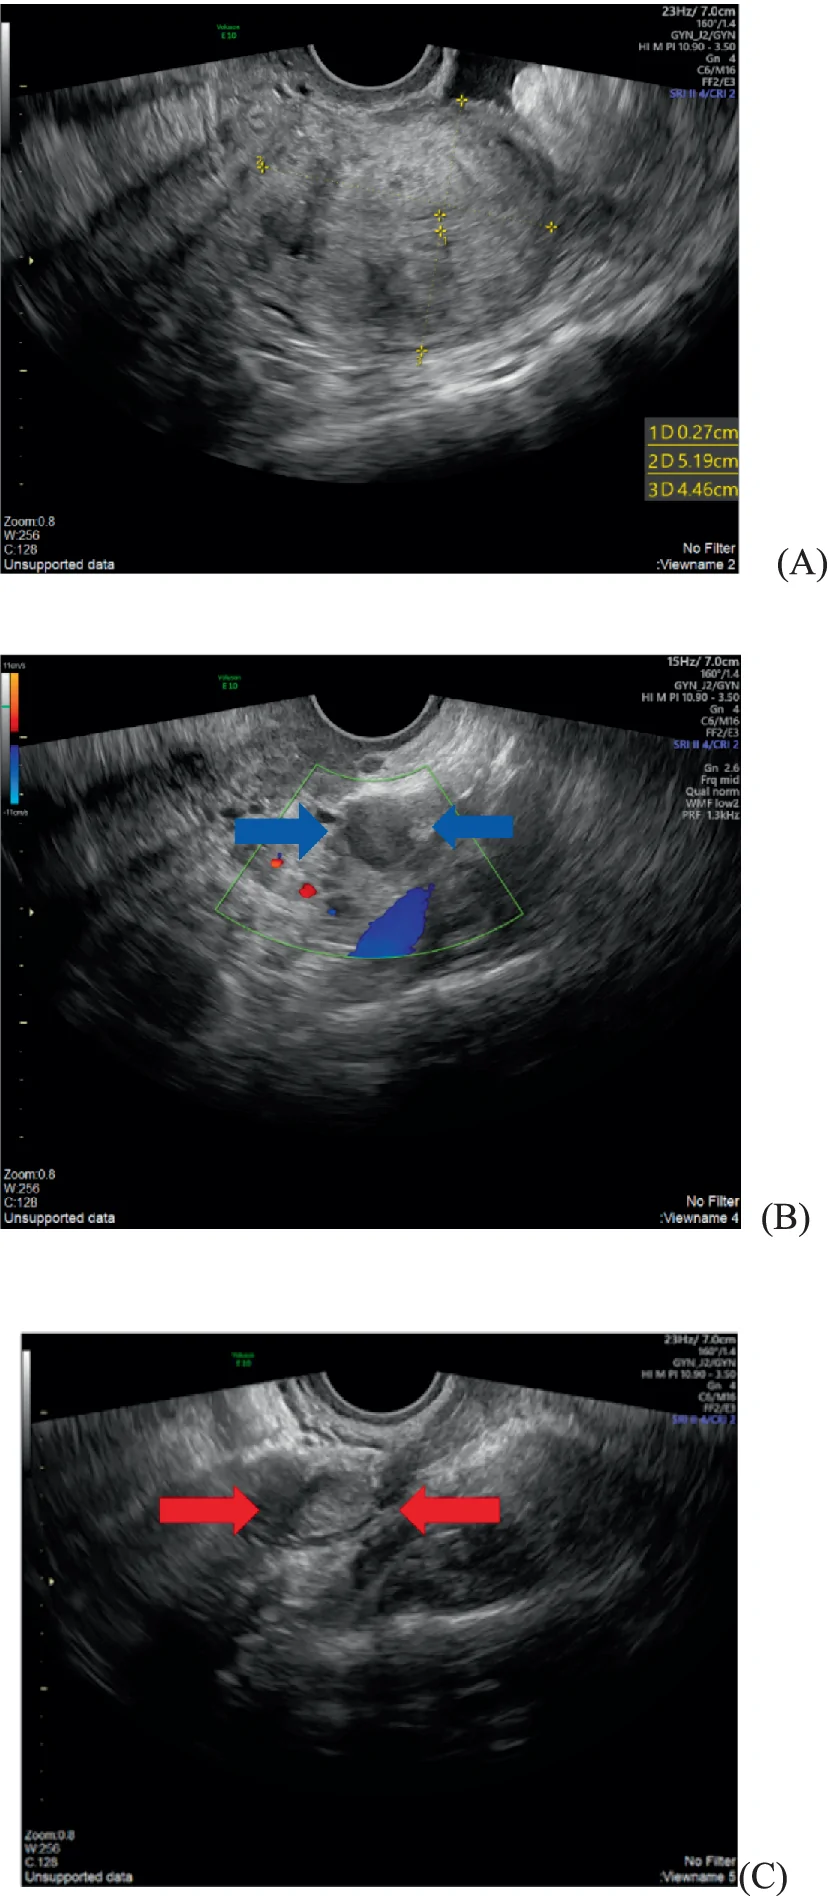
Transvaginal ultrasonography of the uterus demonstrates a measuring 5.19 cm × 4.46 cm diameter. The endometrium is thin and regular, with a measured thickness of 2.7 mm, showing no evidence of intracavitary pathology or focal thickening (A). Ultrasound image of the left ovary (dimensions: 2.58 × 1.79 cm) revealing a hypoechoic lesion measuring 1.26 × 1.14 cm, highlighted by the blue arrow (B). The right ovary measured 2.42 × 1.17 cm, as indicated by the red arrow (C). No antral follicles are visualized in either ovary.
